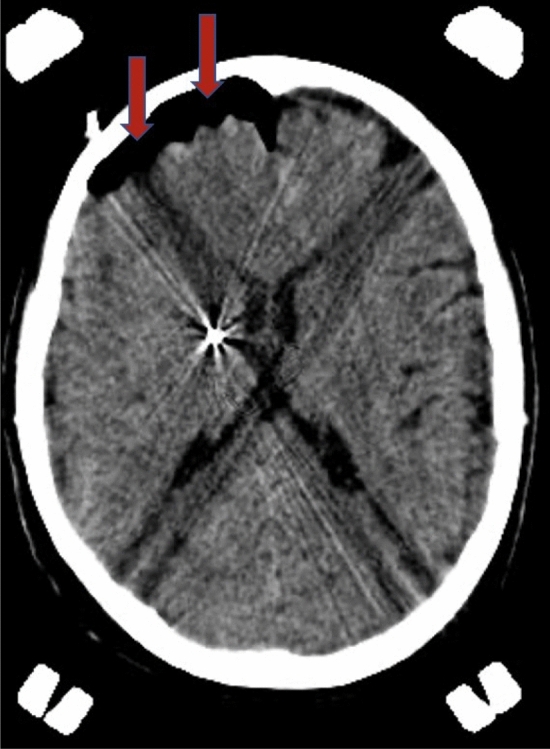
CT scan performed after a unilateral DBS procedure showing the entry of intracranial air (indicated by the red arrows) ipsilateral to the implanted electrode. *CT* computerized tomography, *DBS* deep brain stimulation.
